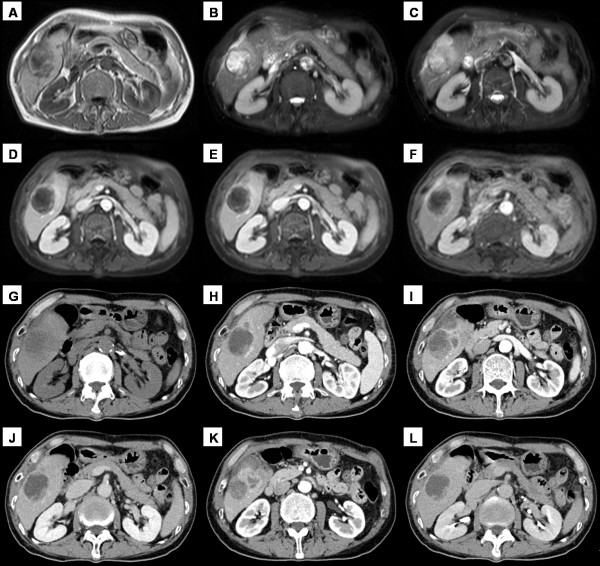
A 63-year-old man with abdominal pain. (A-C) MR images revealed a mass with low signal intensity on T1-weighted imaging and high signal intensity on T2-weighted imaging. (D-F) The periphery of the mass was slightly enhanced during the arterial, portal or delayed phase of contrast enhancement. (G) On CT, a heterogeneous hypodense mass was revealed. (H-L) After contrast material injection, the mass showed gradual, inhomogeneous enhancement, with decreased enhancement on delayed scans.
